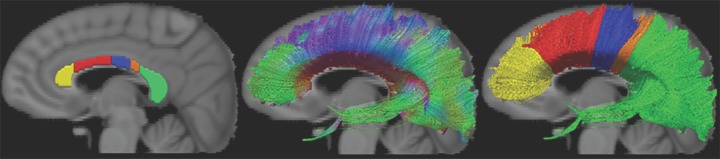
Seed ROIs and example segmentation of the corpus callosum overlaid on an MNI T1-weighted template. The CC was segmented into the following five regions: CC-I (yellow), CC-II (red), CC-III (blue), CC-IV (orange), and CC-V (green). The middle image shows a streamline reconstruction of the CC with DEC encoding based on the primary eigenvector direction. The rightmost image shows the same reconstruction with color determined by the seed ROI from which the tract originated.
